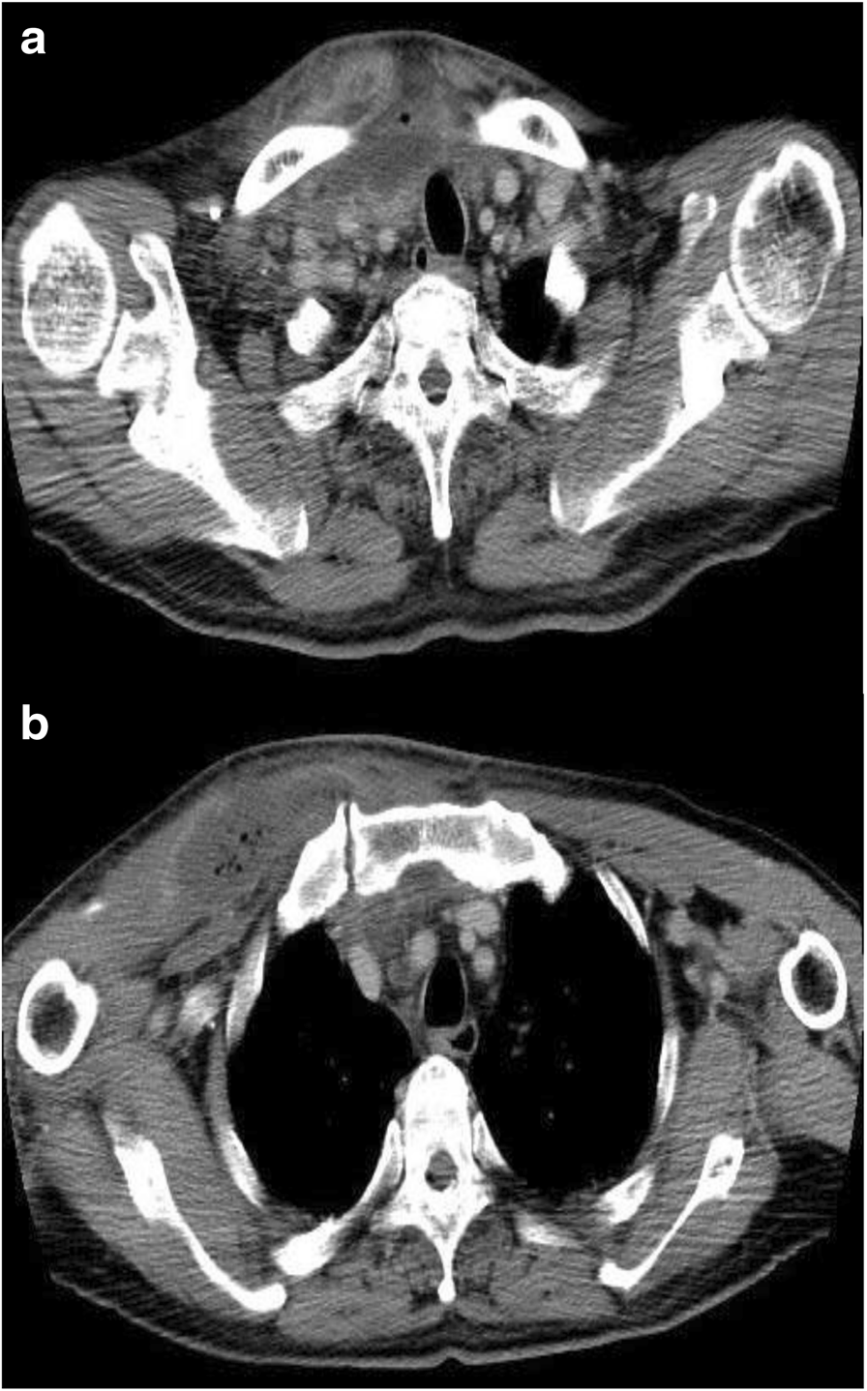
Computed tomographic scans of the chest on admission. Computed tomography of the chest detected an abscess with air located below the thyroid gland and involving the right pectoral major muscle around the right sternoclavicular joint (a, b), as well as disaggregation of the right sternoclavicular joint (b)
